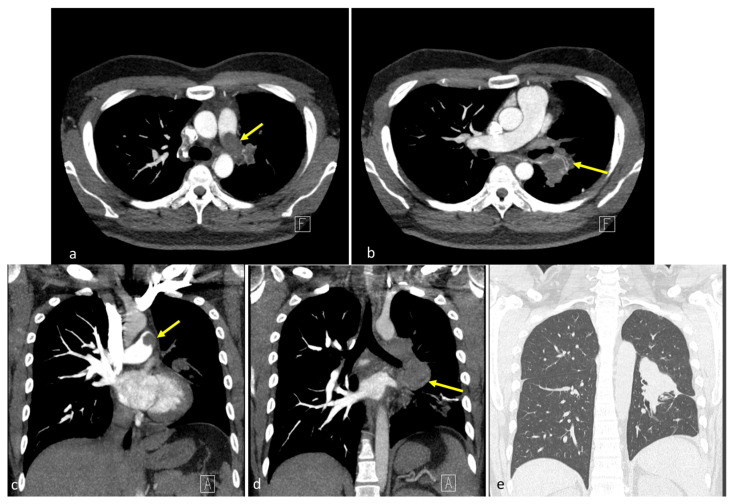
Preoperative computed tomography findings. (a,b) Coronal images revealed a 7.1 cm lobulated, heterogeneous mass spanning the upper left and lower lobes, extending medially into the pulmonary hilum (yellow arrows) [1,2,3]. The left pulmonary artery was encased with intraluminal lobulated filling defects, forming acute angles with the vessel wall, with suggested tumor thrombus. No pleural effusion or mediastinal shift was observed. (c,d) Axial sections showed a centrally located soft tissue mass with irregular margins involving the left hemithorax, with mild post-contrast enhancement and direct invasion into the left pulmonary artery. Intraluminal filling defects contiguous with the mass were identified. (e) Regarding lung window settings, the mass appeared as a dense, ill-defined opacity occupying the central left hemithorax, merging into the adjacent lung parenchyma. The letters “A” and “F” on the images represent standard orientation markers indicating anterior (A) and foot (F) directions, respectively.
